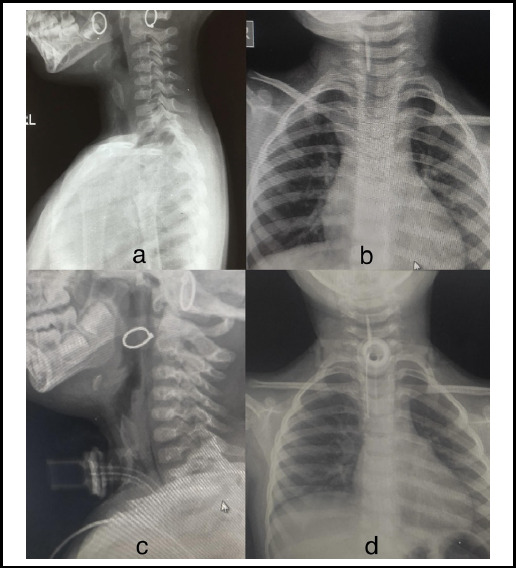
X-ray showing Subglottic FB: Before tracheostomy a) Antero-posterior b) Lateral view and After tracheostomy c) Antero-posterior d) Lateral view.
